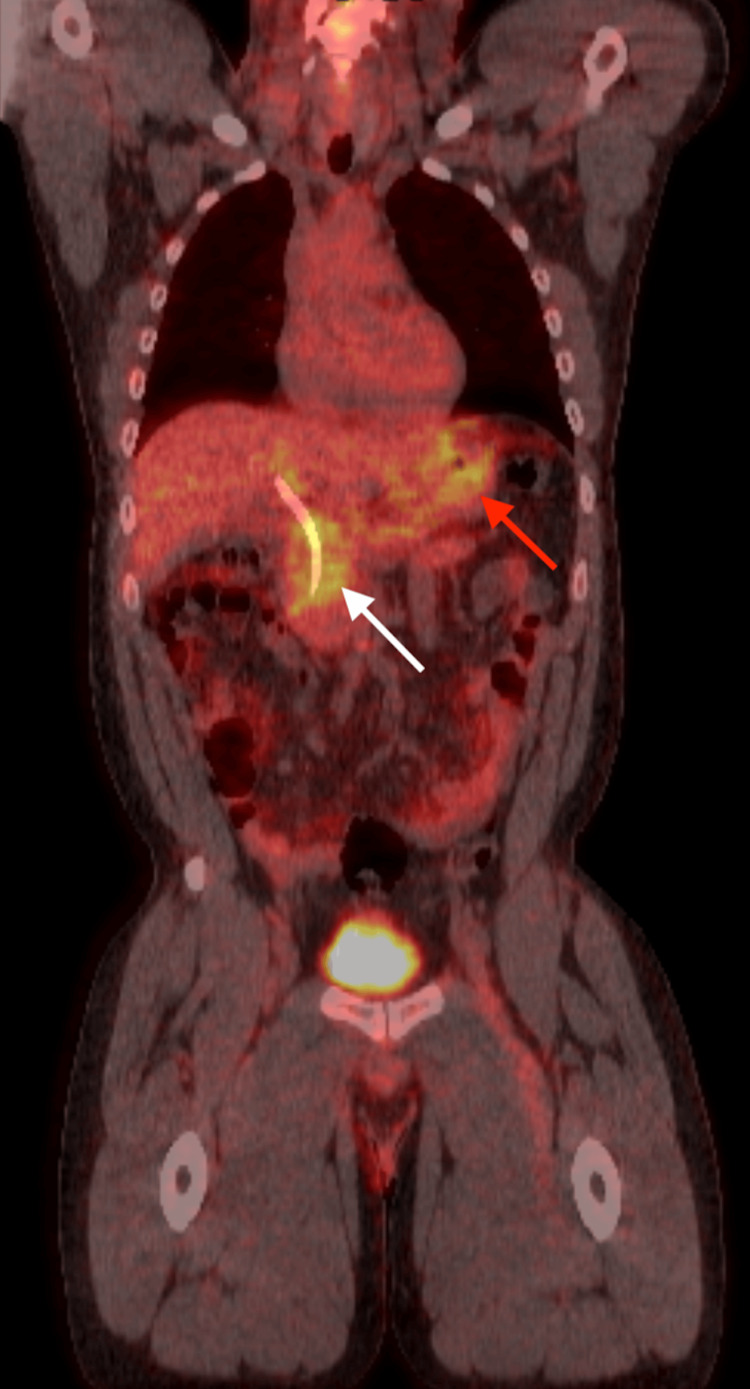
Positron emission tomography (coronal view) demonstrating abnormally elevated F-FDG uptake in the pancreatic head (white arrow) and gastrum (red arrow).
